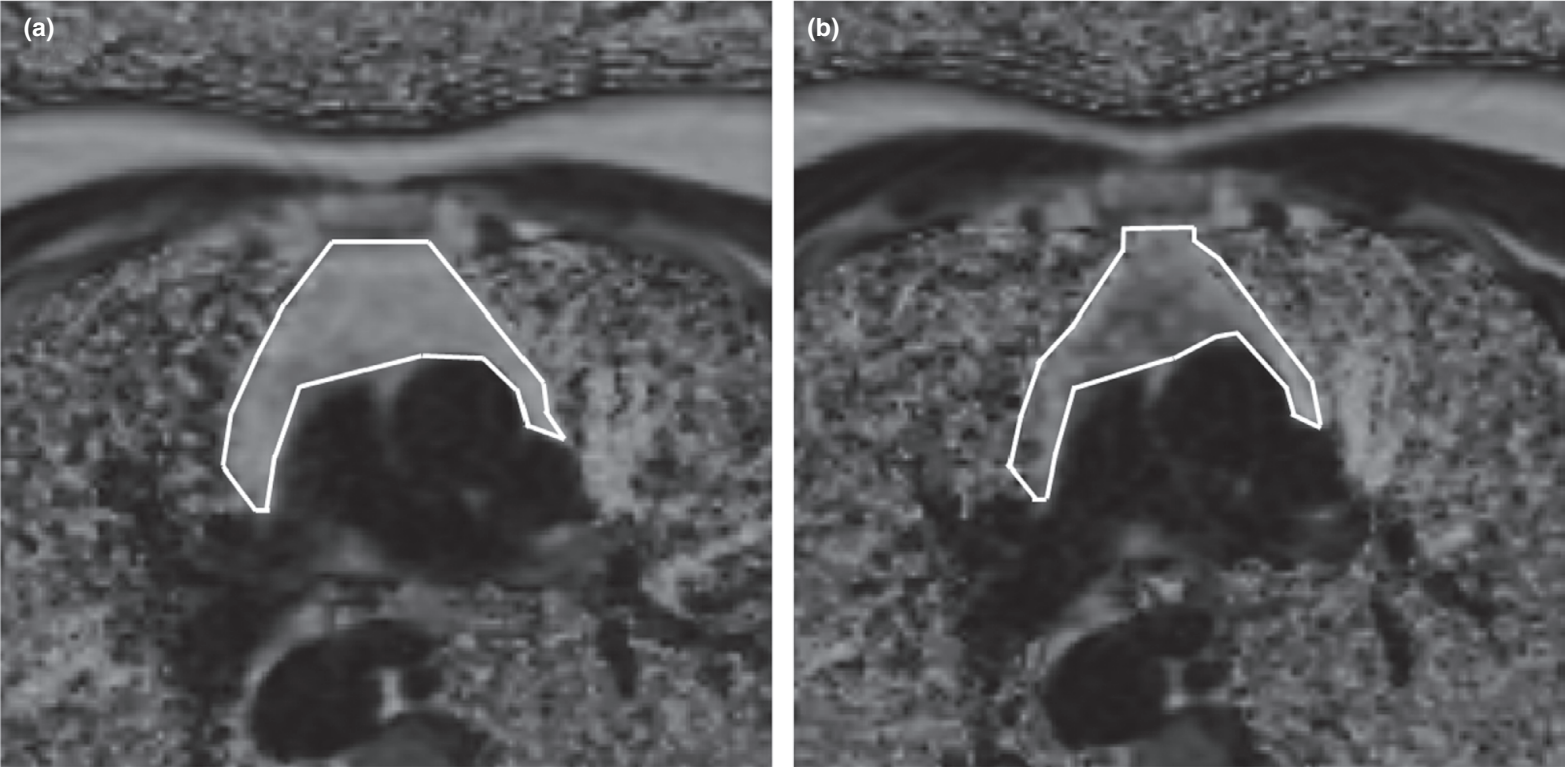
Example of treatment‐induced change in thymic MRI appearance. Darkening corresponds to replacement of fat with nonadipose tissue. White lines denote the thymic boundary. Volunteer 2 at 0 (a) and 9 (b) months
